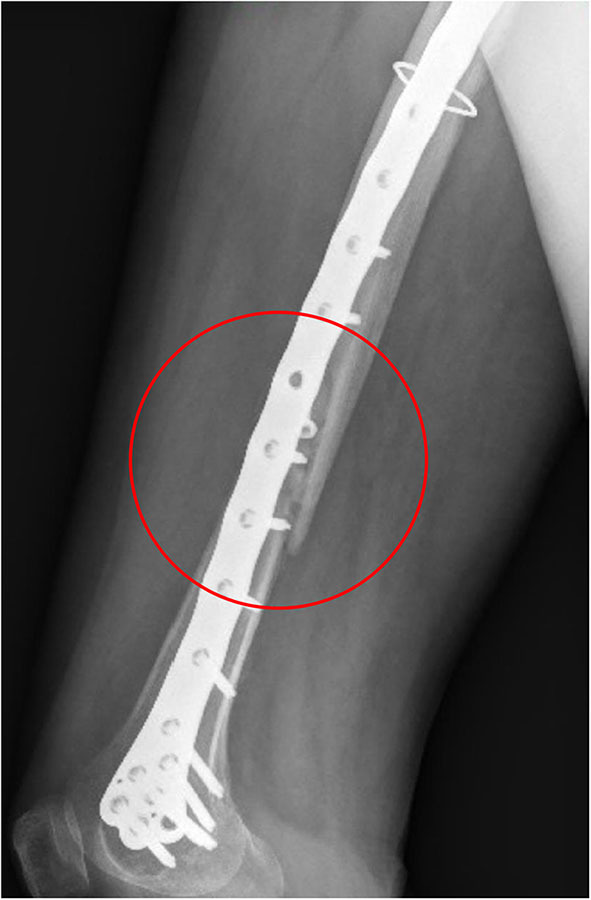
X-ray image taken after 2 months from the surgery. The non-consolidation of the bone fracture is pointed out.
